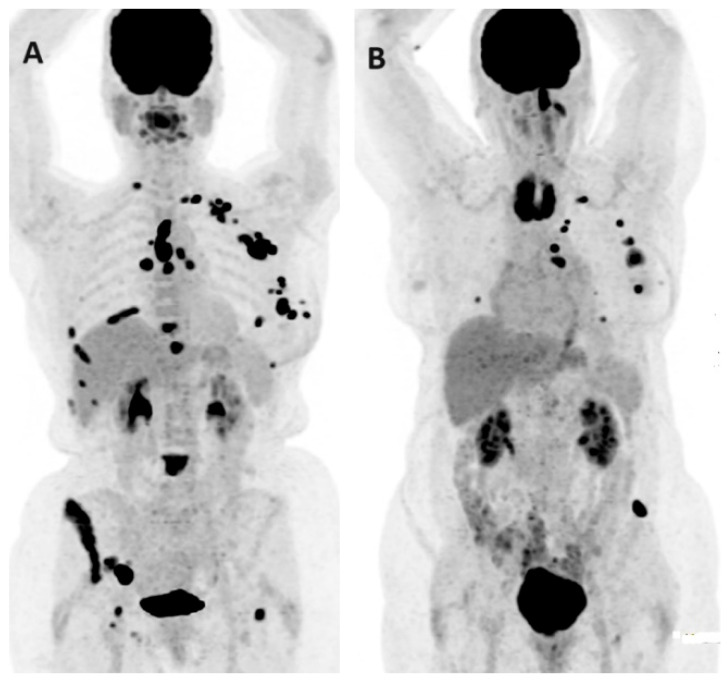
FDG PET/CT in BC initial staging. PET/CT MIP images of two breast cancer patients (A,B) demonstrate intensely FDG-avid multifocal left breast cancer, with an unexpected extensive burden of regional and non-regional nodal disease as well as multifocal distant osseous and pulmonary metastases.
